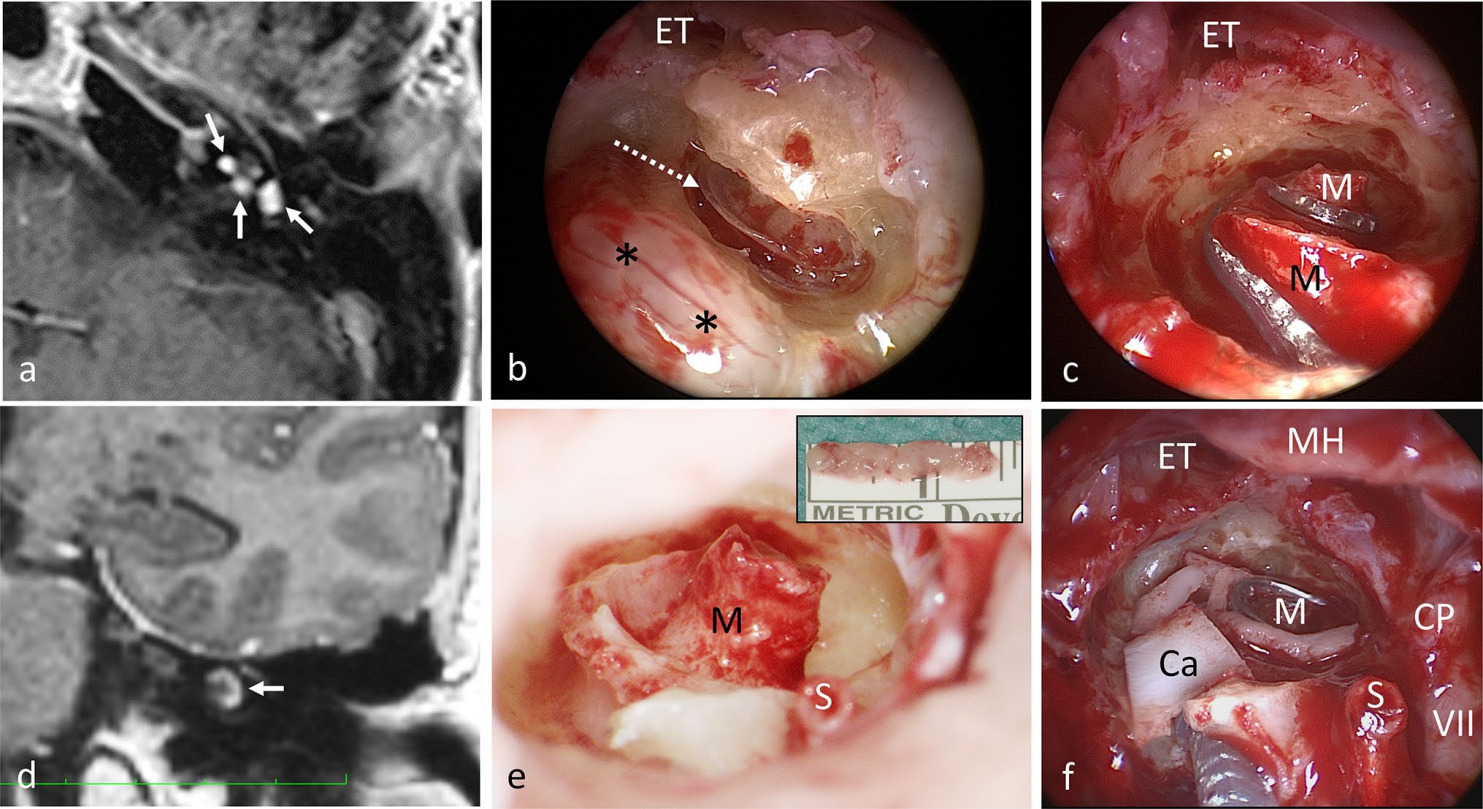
a MRI of pat. #1 (T1-w with contrast medium, axial) showing the tumor (→) in the cochlea, in the vestibule and in the fundus of the internal auditory canal. d MRI of pat. #2 (T1-w with contrast medium, coronal) demonstrating a solely intracochlear tumor (→) in a young patient. b, c, e, f Intraoperative views of patients #1 (b, c) and #2 (e, f). The intracochlear tumor parts of patient #1 can be seen in the basal turn (*) while the second turn is tumor free (b). The tumor from patient #2 is shown  after removal in the insert in e. The perimodiolar formed electrode array was placed around the preserved basal and second turn modiolus (M). f Cartilage chips (Ca) were placed peripheral to the electrode array and the defect was closed with a cartilage-perichondrium-island transplant (not shown). Dotted arrow in b basilar membrane, *VII* facial nerve, *CP* cochleariform process, *ET* Eustachian tube orifice, *MH* Malleus handle, *S* stapes head, *w* weighted
